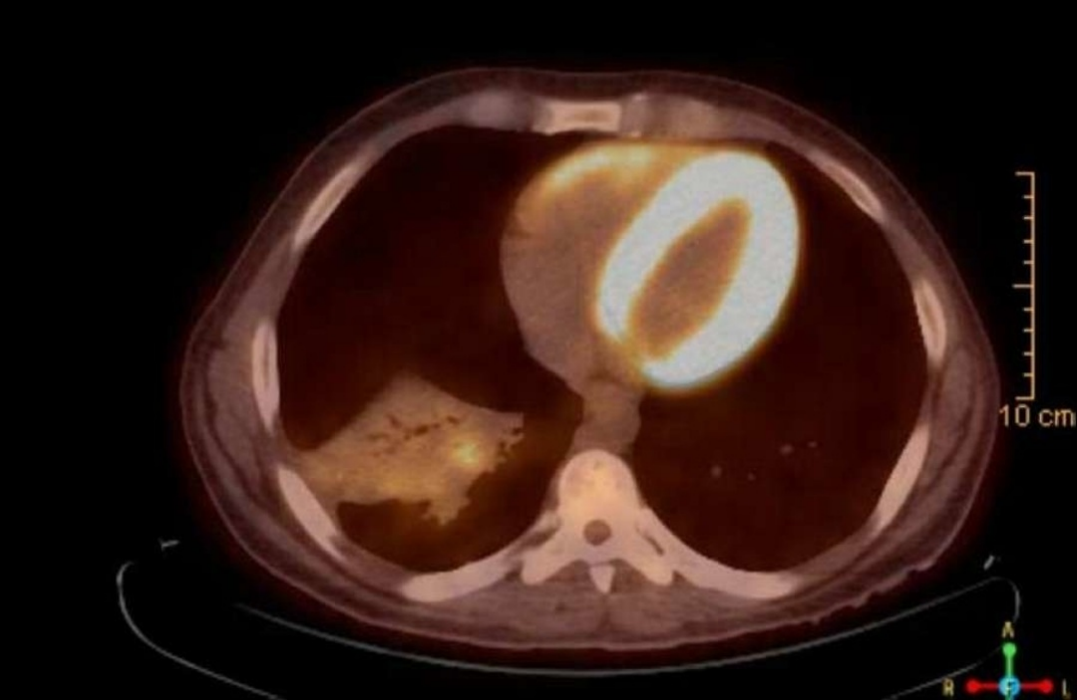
Ill-defined FDG-avid mass measuring 9.5 x 5.0 cm involving the right lower lobe demonstrating a standardized uptake value (SUV) max of 4.5 on PET/CT, concerning for malignancy. FDG: 2-[18F]-fluoro-2-deoxy-D-glucose
